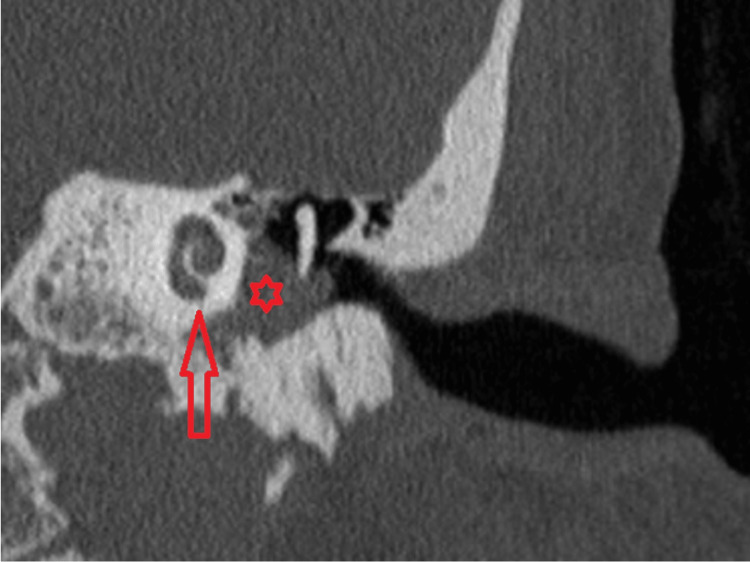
High-resolution coronal CT IAC without contrast showing the left middle ear filled with soft tissue CT: computed tomography; IAC: internal auditory canal
